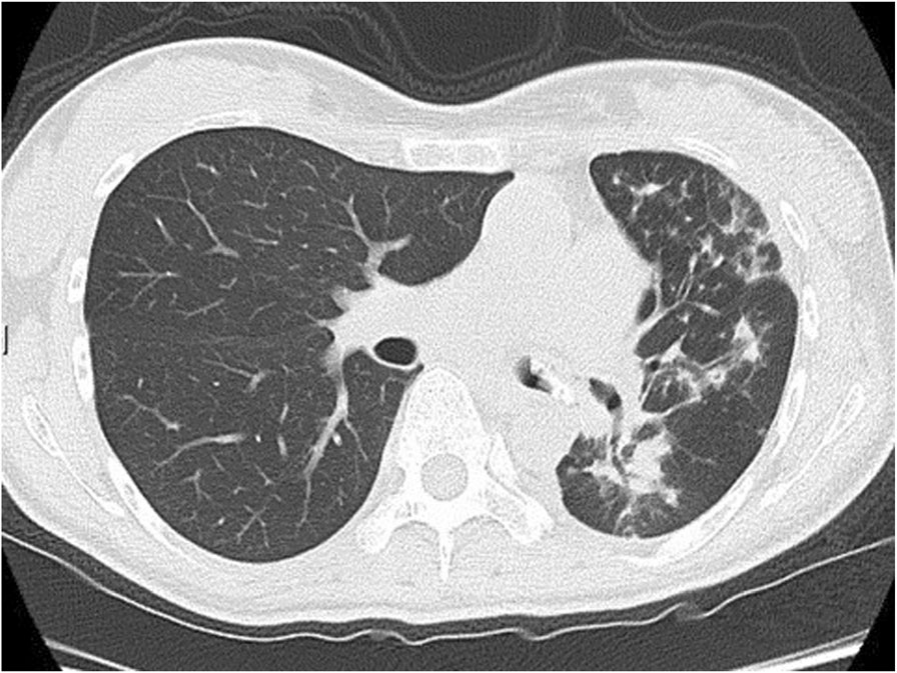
Axial chest computed tomography (CT) images at admission show a mass surrounding the patient’s left lower bronchus stump with endobronchial extension causing significant obstruction of the left upper bronchus
